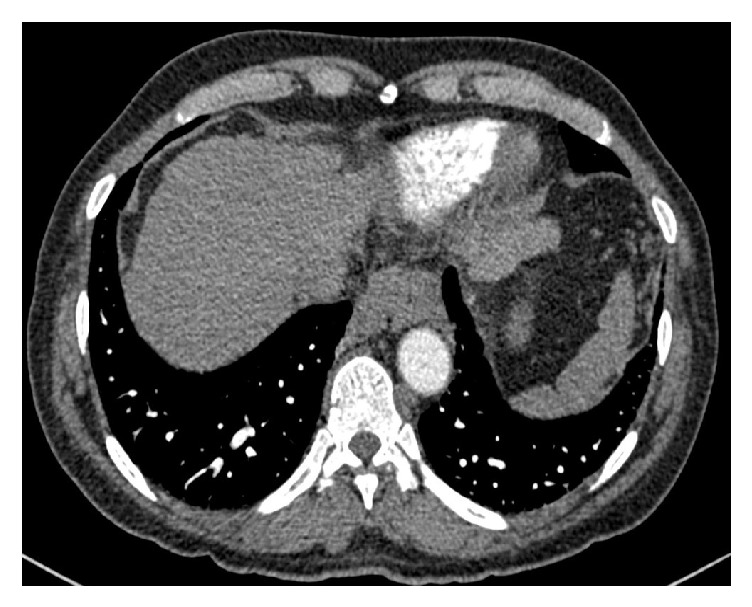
Chest CT scan showing the prominent pulmonary vasculatures and irregular borders of the liver.
